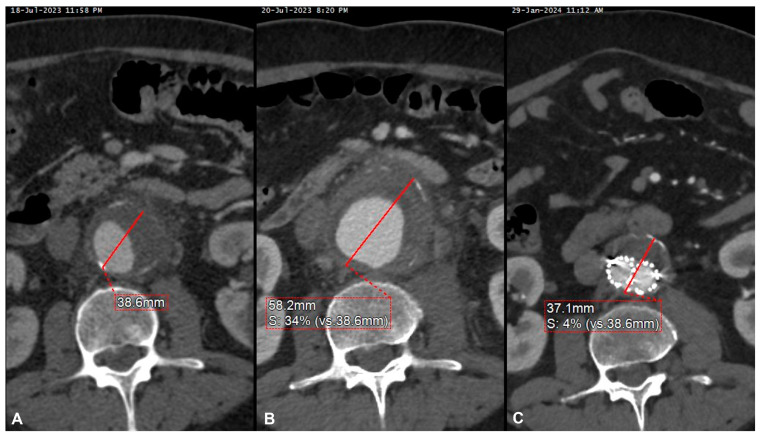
Pre-operative computed tomography angiography (CTA) of symptomatic small abdominal aortic aneurysm (A) and 2-day CTA performed for persistent pain showing a 20 mm aneurysm’s growth (B), urgently treated with EVAR with a good clinical and radiological result, as demonstrated by the significant aneurysm’s shrinkage noted at the 6-month postoperative CTA (C).
